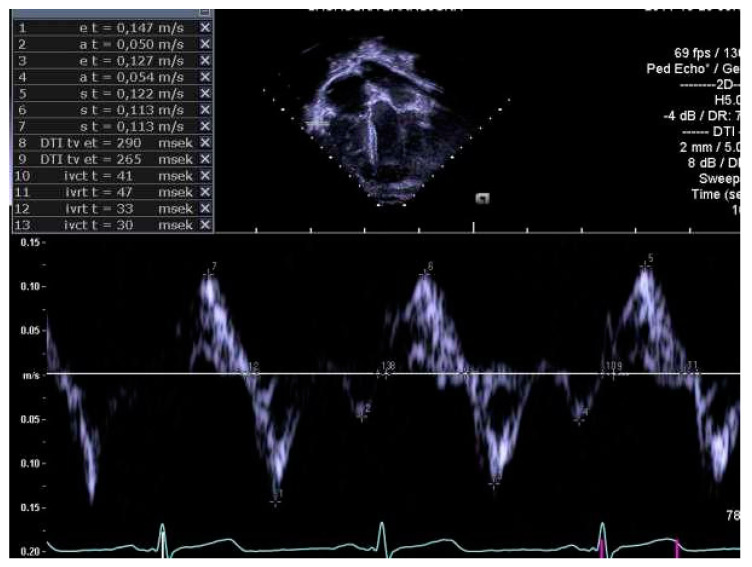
Right ventricular myocardial performance index (RVmpi’) assessed by pulsed wave tissue Doppler of right ventricular free wall.
